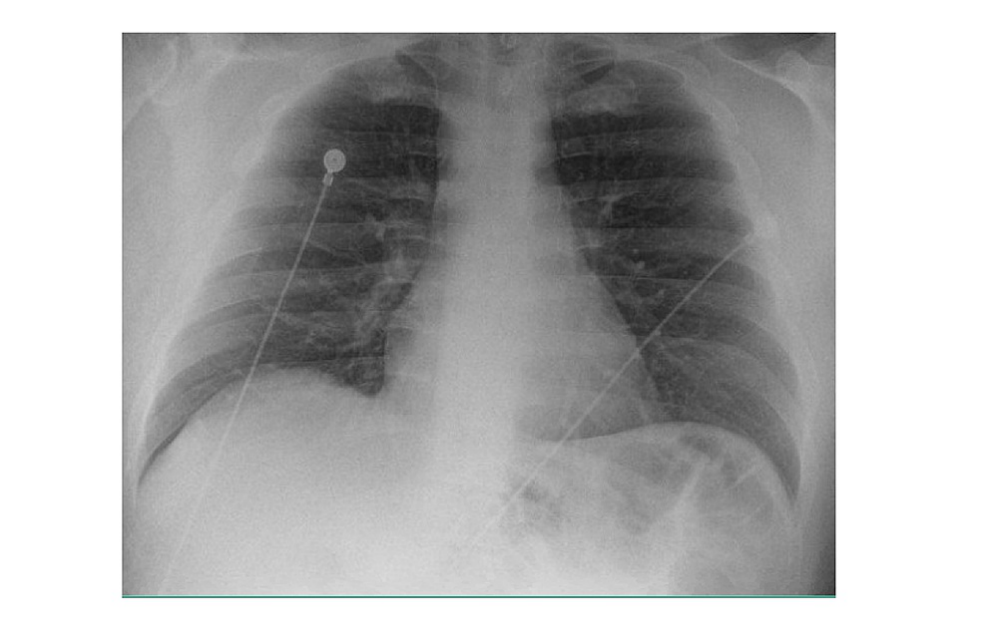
Chest X-ray (anterior-posterior view): no evidence of cardiopulmonary abnormality
